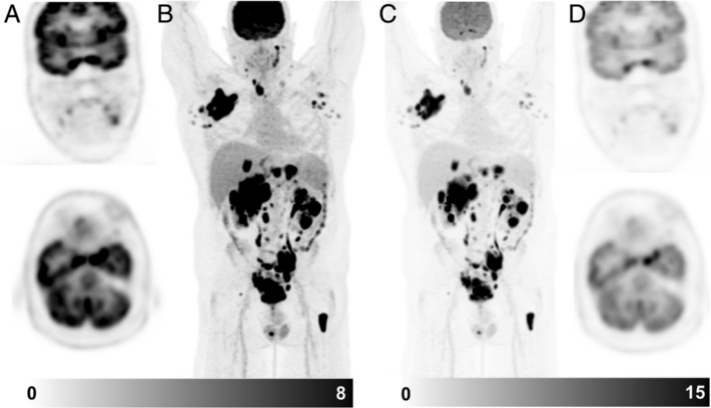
Patient with diffuse large B cell lymphoma. On the standard windowing, no abnormality is readily identified in the brain (a coronal & axial slice, b MIP image). By increasing the upper SUV threshold, abnormal uptake becomes readily becomes visible (c MIP image, d coronal & axial slice). This corresponded to a MRI abnormality which was not reported prospectively but identified following targeted review after the PET scan. Changing the PET window so that abnormalities can be identified above physiologic brain activity should be a routine component of image review
